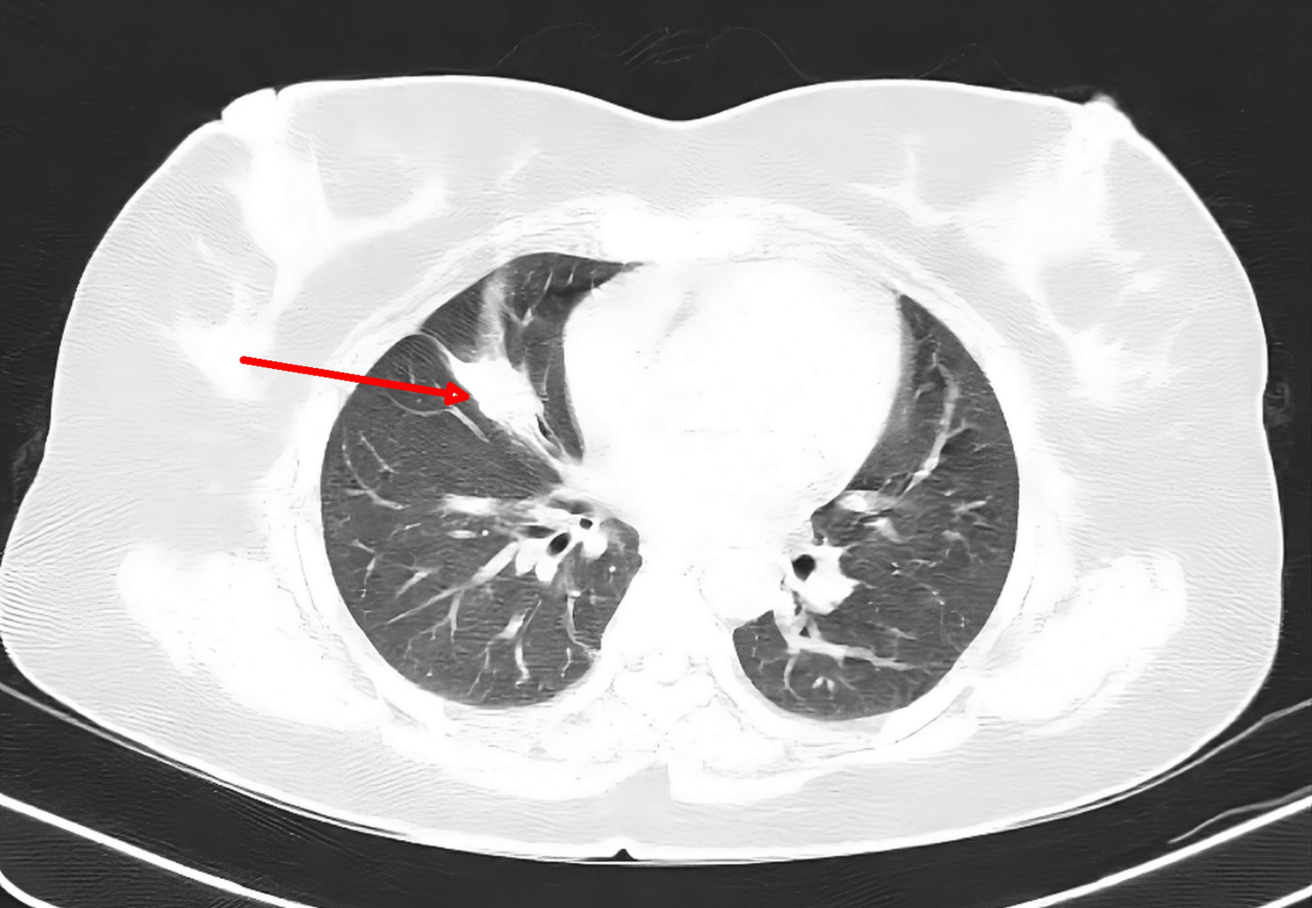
HRCT thorax showing segmental atelectasis HRCT: High-resolution computed tomography
